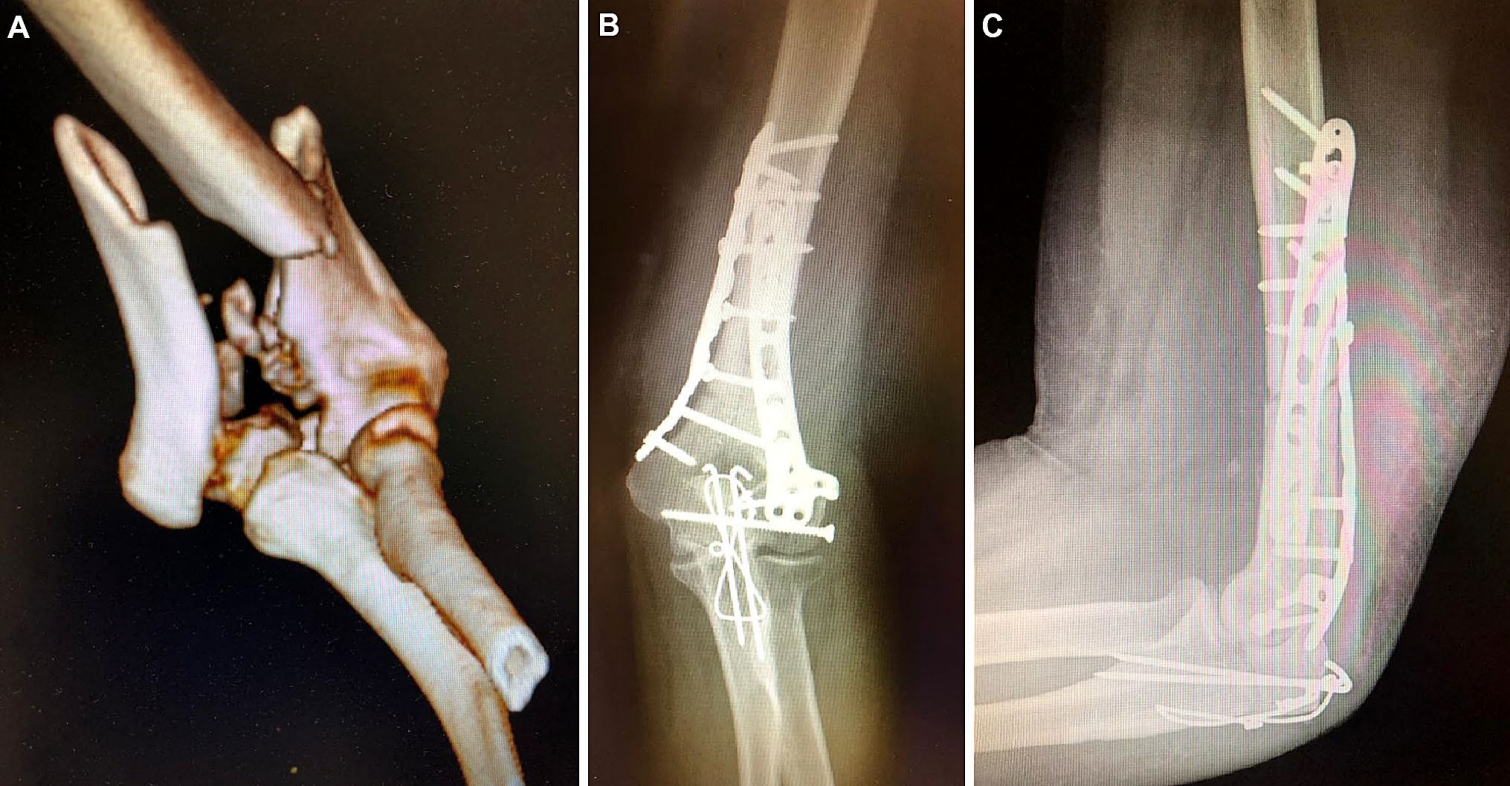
a Initial 3D CT-scan of a 71-year-old female patient, having suffered an AO 13 C3 fracture of her left arm by a fall from standing height. b, c Final follow-up X-rays after 2 years with stable fixation and bony healing
